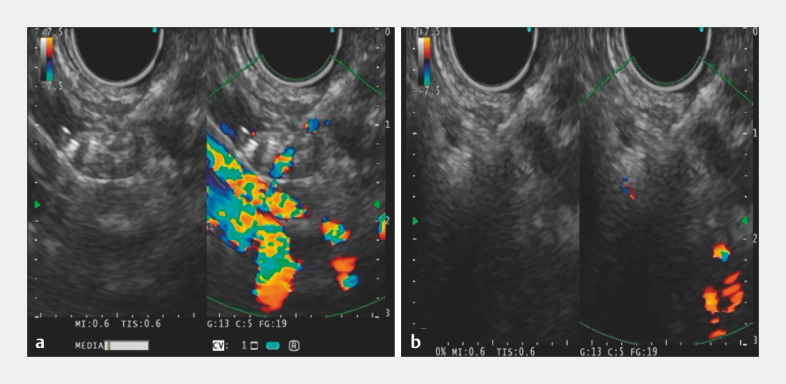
Endoscopic ultrasound (EUS) images showing:
